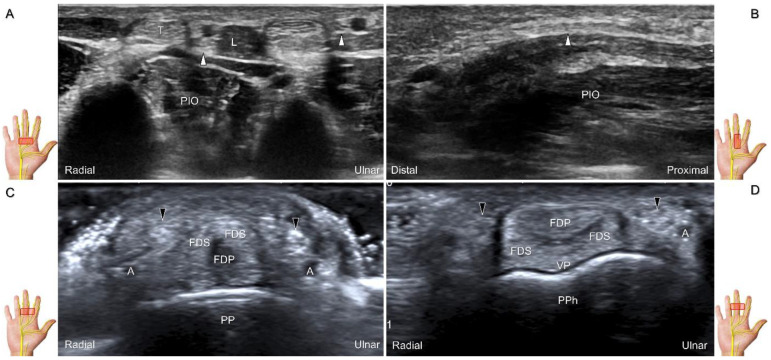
Sonographic imaging of the palmar common digital nerves in short-axis (A) and long-axis (B) views. Palmar proper digital nerves from the base (C) to the head of the proximal phalanx (D). White arrowheads: palmar common digital nerves; black arrowheads: palmar proper digital nerves. T: flexor tendon; L: lumbricalis muscle; PIO: palmar interosseous muscle; FDS: flexor digitorum superficialis tendon; FDP: flexor digitorum profundus tendon; A: artery; VP: volar plate; PP: proximal phalanx; PPh: head of the proximal phalanx.
